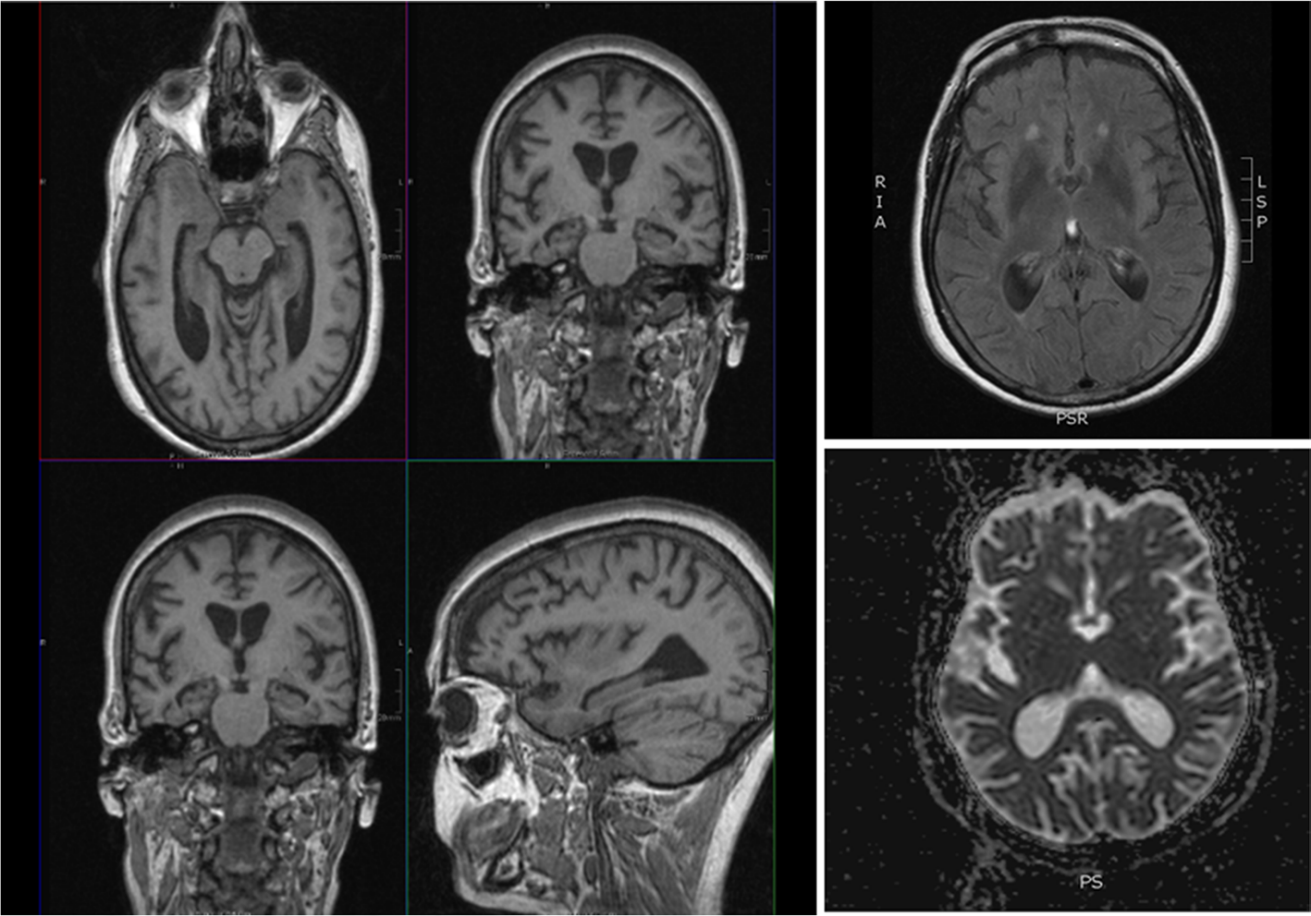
Magnetic resonance imaging scan of the index patient
